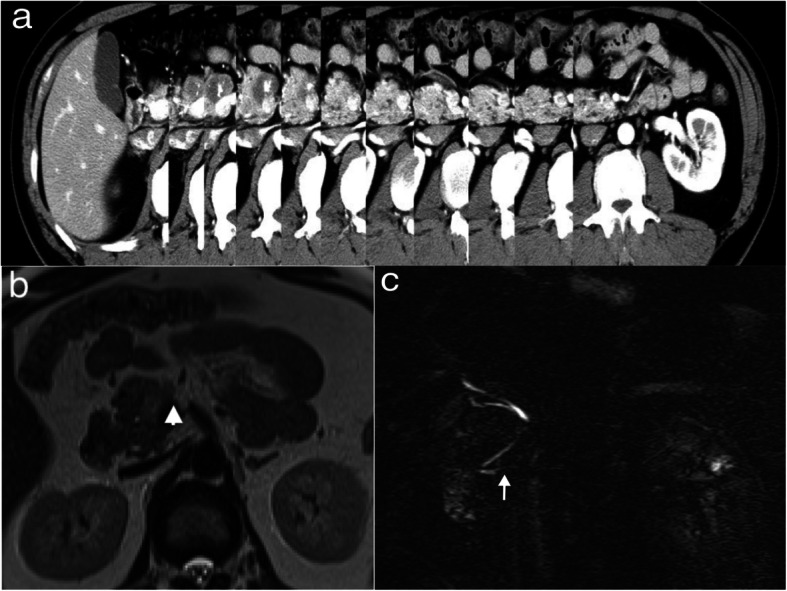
Abdominal contrast-enhanced computed tomography and magnetic resonance imaging findings*.*a Abdominal contrast-enhanced computed tomography scan showing a mass measuring 30 mm in diameter with calcification, which projected forward in the pancreatic head without the body and tail. b T2-weighted magnetic resonance imaging scans showing a high signal intensity in the body and tail of the pancreas (arrowhead). c Magnetic resonance cholangiopancreatography image showing complete disruption of the pancreatic duct of the body and tail (arrow)
